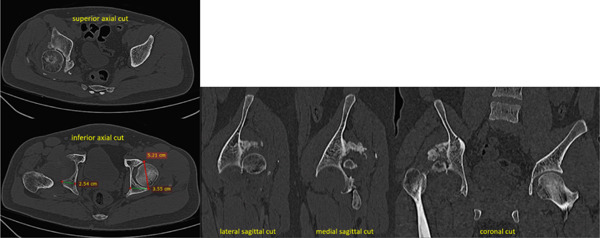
Case 1 preoperative computed tomography.
